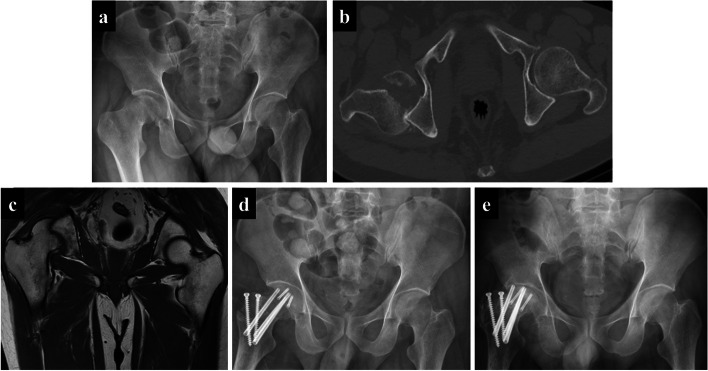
A representative case of the second subtype of iFHNF, namely posterior dislocation and fracture of the femoral head with subsequent femoral neck fracture. A 42-year-old man involved in a severe traffic accident was diagnosed with femoral head fracture and posterior hip dislocation based on X-ray (a) and CT images (b). No visible indications of bone fractures were observed in the femoral neck on MRI images (c). However, the femoral neck was fractured during open reduction and internal fixation. Thus, the femoral head and neck were internally fixed (d). Finally, femoral head necrosis was detected (e)
